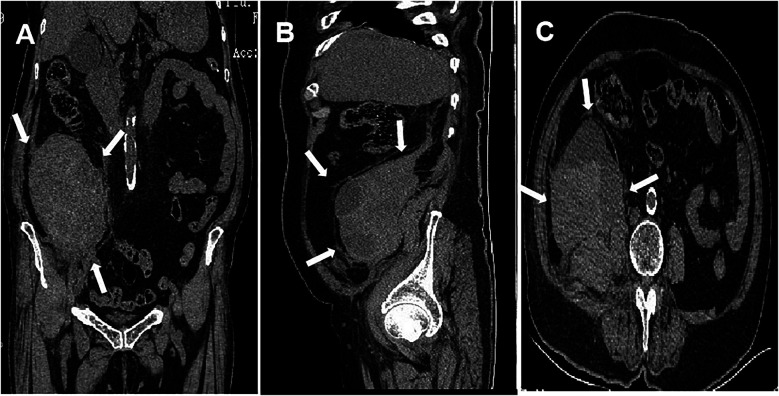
Abdominal CT imaging demonstrating left retroperitoneal hematoma formation (arrows). (A) Coronal plane; (B) sagittal plane; (C) cross-section.
